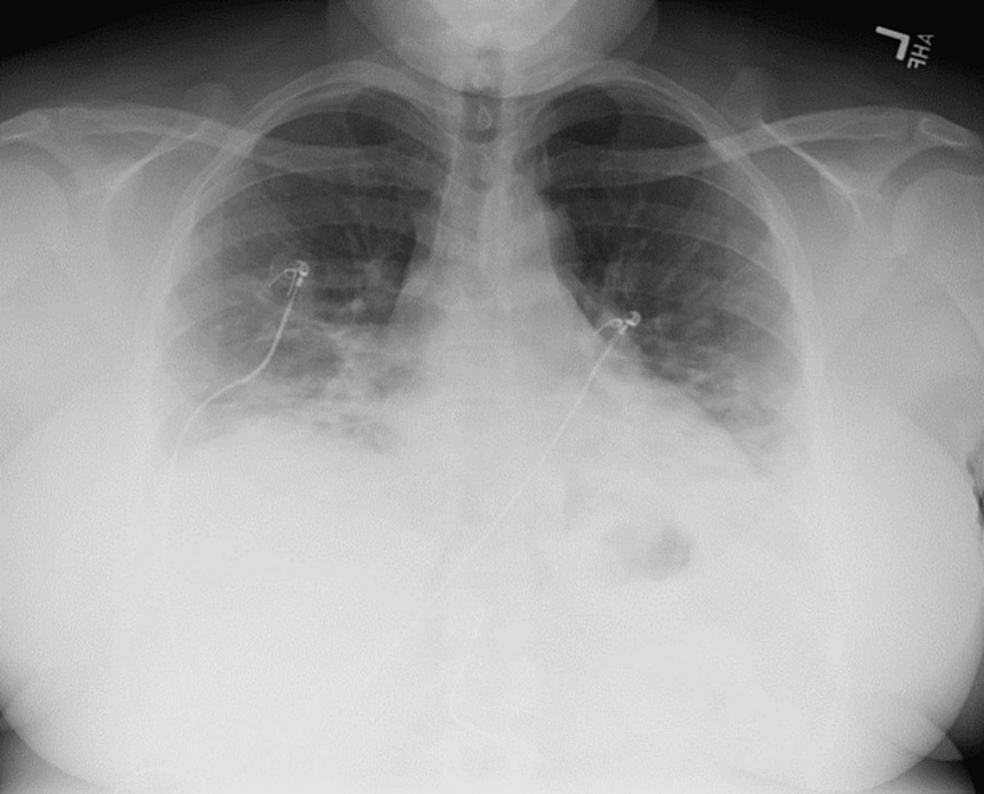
A chest X-ray taken five days after the initial X-ray revealed extensive bilateral airspace disease with a differential of pulmonary edema versus multifocal pneumonia
